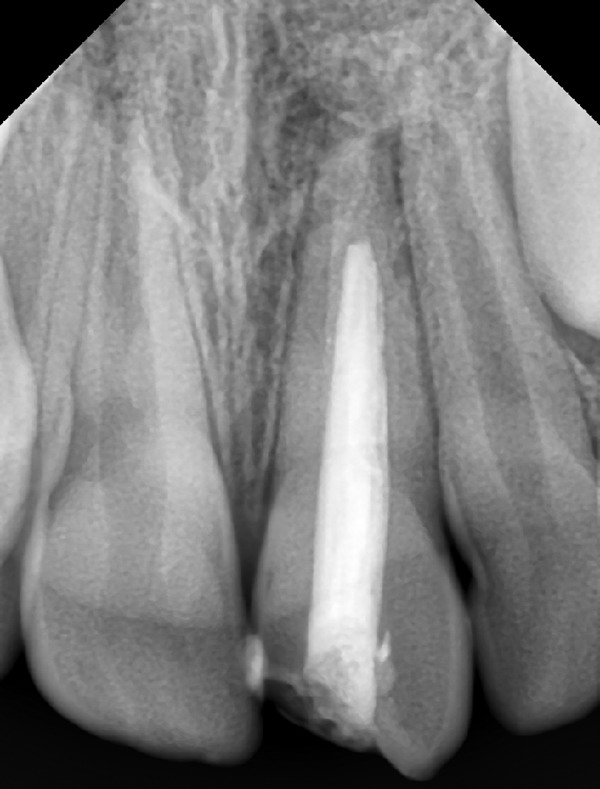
Intraoral periapical view showing obturation of the canal with gutta-percha
